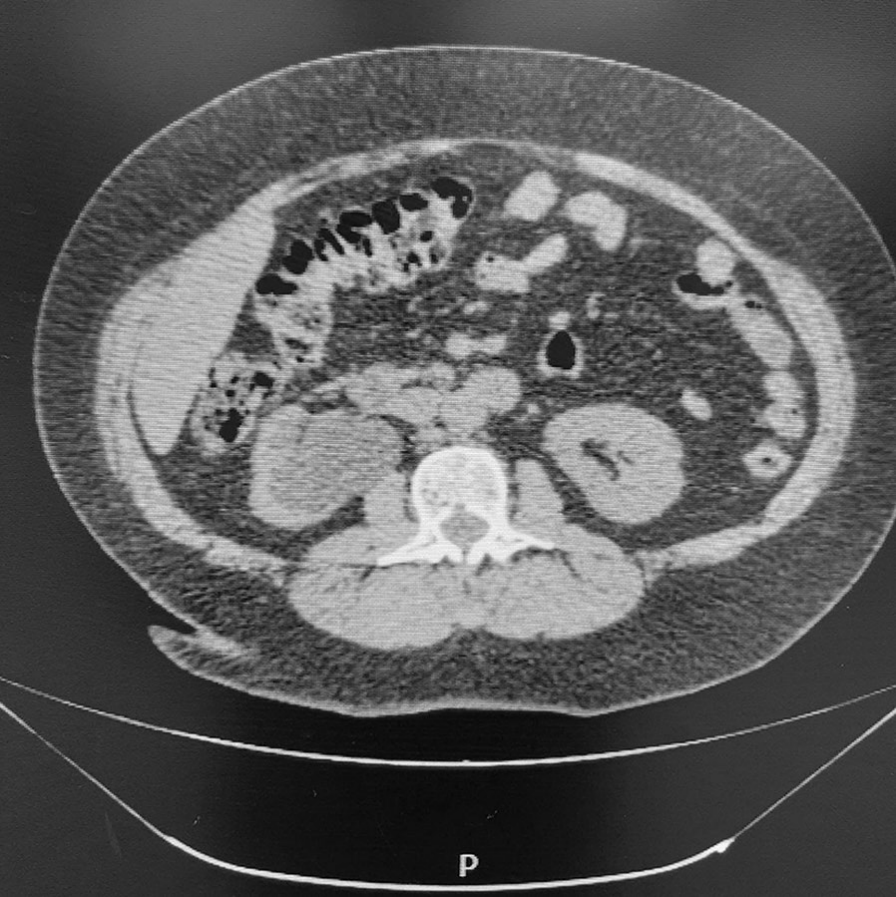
Noncontrasted CT with right hydroureteronephrosis
